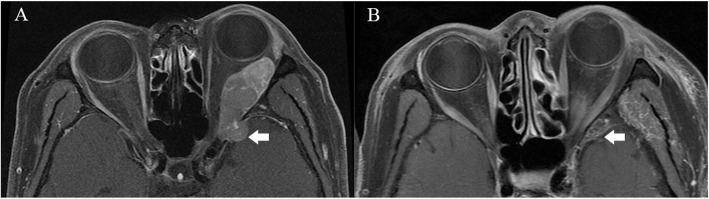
Case 2. The patient was a 45-year-old with a chronic disease process, left exophthalmos, and vision loss for more than 2 years. a: MRI showed that the main body of the tumor was located lateral orbit and invaded the intracranial dura through the superior orbital fissure (arrow). b: After the Transfronto-orbital approach was given to the craniotomy, the MRI re-examinations immediately after the operation showed that the tumor and involved dura were removed completely (arrow). There was no recurrence after 8 years of MRI follow-up
